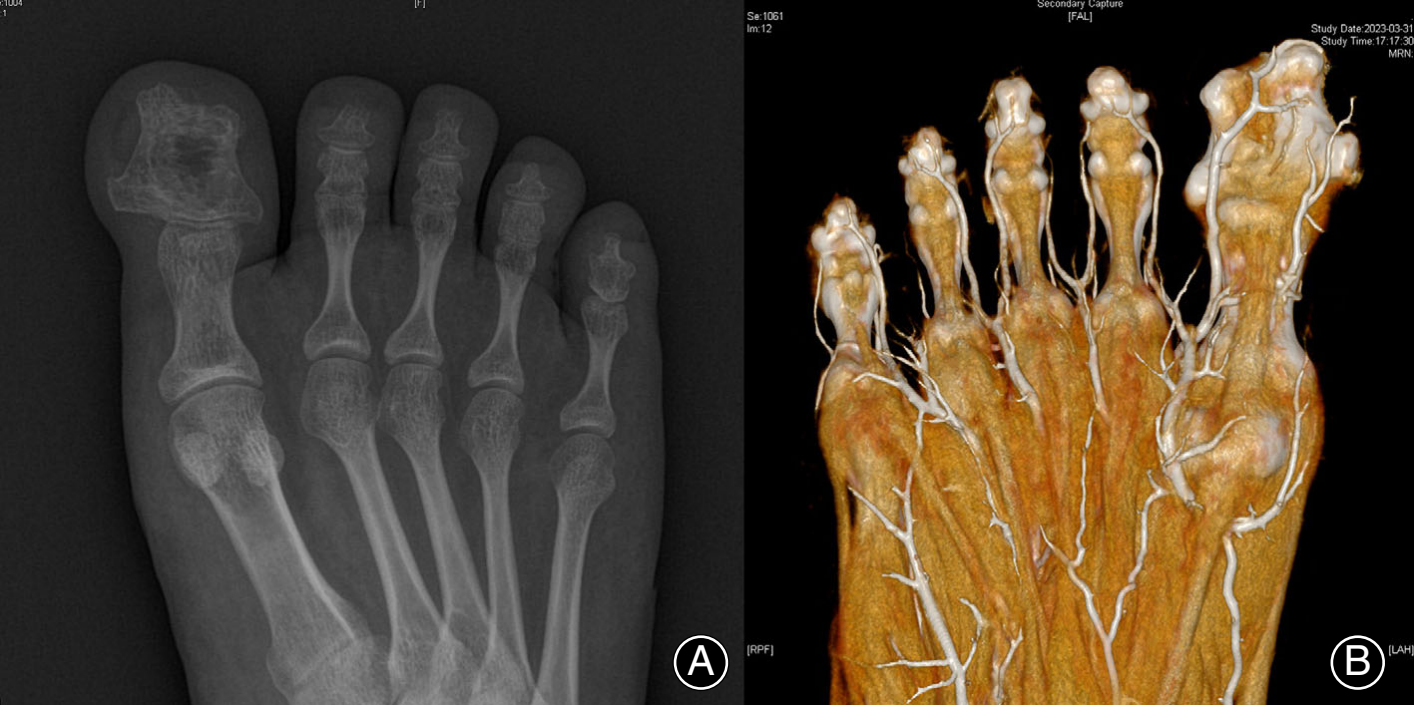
(A) X‐ray and (B) CTA of the great toe after transverse enlargement.
